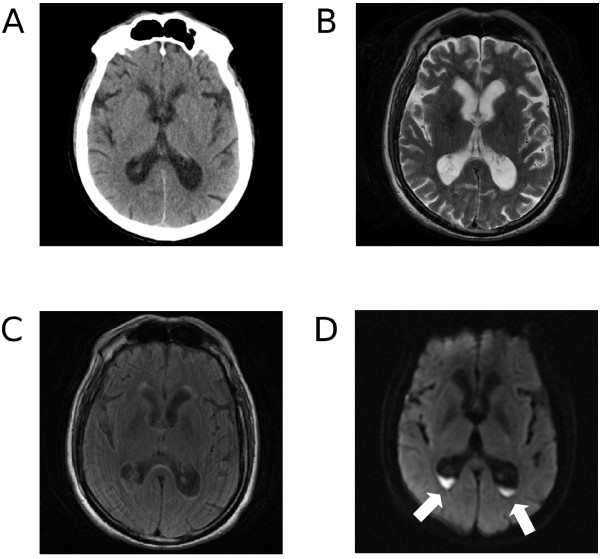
Brain neuroimaging studies performed on the day when the diagnosis of ventriculitis was made. Only the diffusion sequences clearly show the intraventricular debris. A. computed tomography scan; B. magnetic resonance T2 sequence; C. magnetic resonance FLAIR sequence; D. magnetic resonance diffusion sequence. Purulent material inside the ventriculi is indicated by the arrows.
